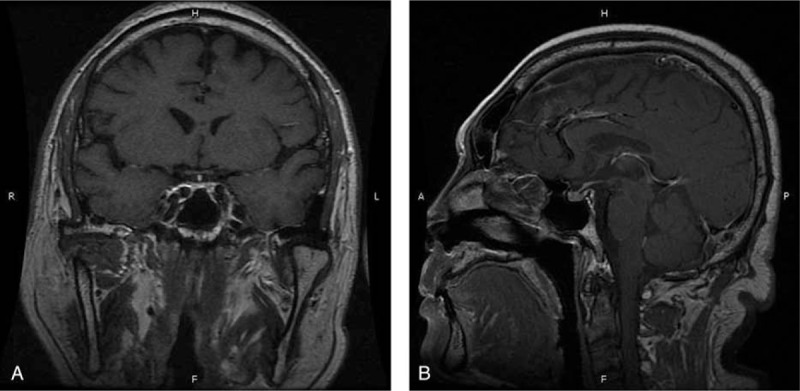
Magnetic resonance imaging (MRI) scan showed heterogenous enhancement of the pituitary gland and enlargement of the basilar part, no space-occupying lesions in the pituitary gland or hypothalamus.
